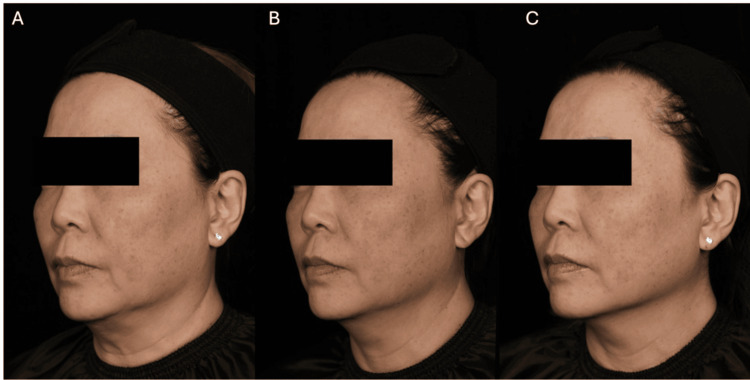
Patient case photographs courtesy of Dr. Tuck Wah Siew. A 58-year-old female with ptosis (jowls), skin laxity and textural abnormalities, facial volume loss, and dyschromia before (A) treatment and three months after receiving a total of 775 MFU-V lines delivered with a 1.5 mm transducer (B), showing visible lifting. Diluted CaHA-CMC (one syringe) was also injected at three months. At a further three months post-CaHA-CMC (C), additional CaHA-CMC and MFU-V-associated lifting and improvements were visible. Written informed consent to include this image in an open-access article was obtained from the patient. CaHA-CMC: calcium hydroxyapatite-carboxymethylcellulose; MFU-V: microfocused ultrasound with visualisation
